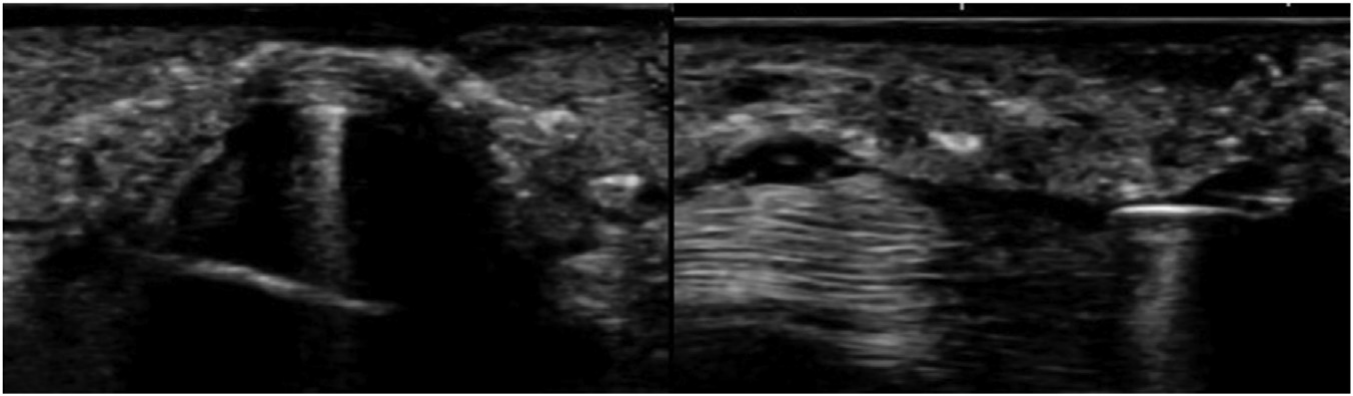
Ultrasonography within the tendon sheath. The left figure shows a short-axis ultrasound image. The guide within the tendon sheath and the neurovascular bundles on both sides of the tendon sheath are visible. The figure on the right shows the long-axis view. The guide is visible in the tendon sheath.
